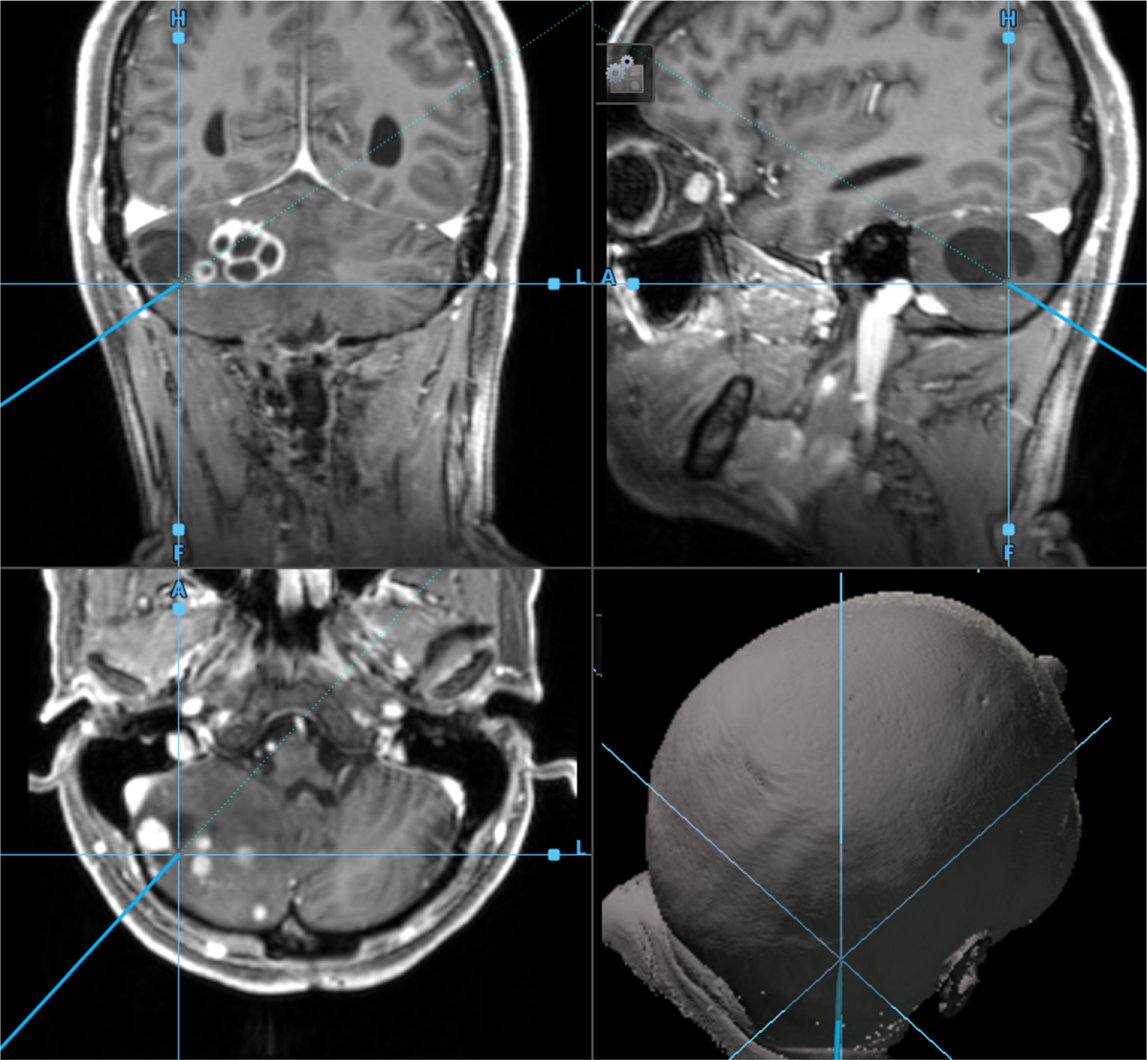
Intraoperative 3D neuronavigation. A preoperatively obtained, high-resolution MRI scan can be used for intraoperative 3D neuronavigation in triplanar visualization. The oblique turquoise line corresponds to a navigation pointer inserted into the posterior fossa, which harbors multiple hemangioblastomas in solid and cystic formation
